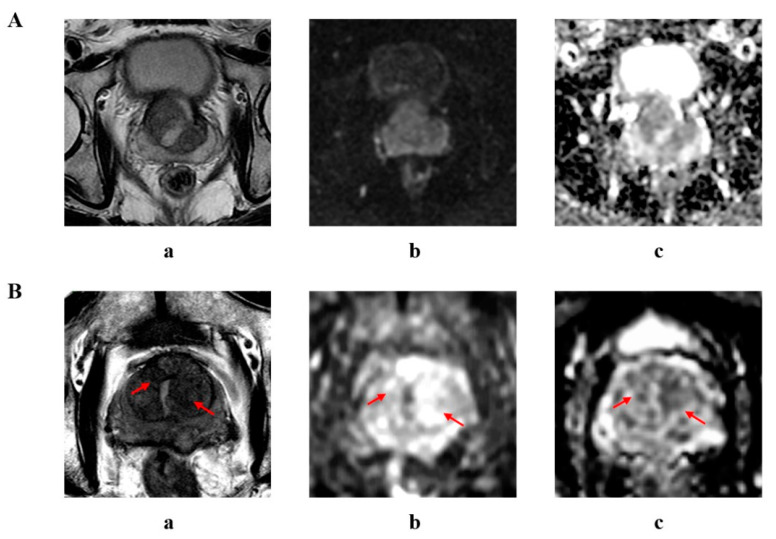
mpMRI results of two biopsy patients with TURP history. (A) A 63-year-old patient previously received TURP; no lesions with PI-RADS score ≥ 3 were found in TZ. Biopsy results showed CSPCa with a Gleason score of 4 + 3 = 7 in the TZ. (B). A 74-year-old patient previously received TURP; two lesions classified as PI-RADS score 4 were found in the bilateral TZ (arrow). Biopsy results were negative in the TZ. (a) T2-weighted image; (b) DWI with b-value of 1500 s/mm^2^; (c) ADC map.
